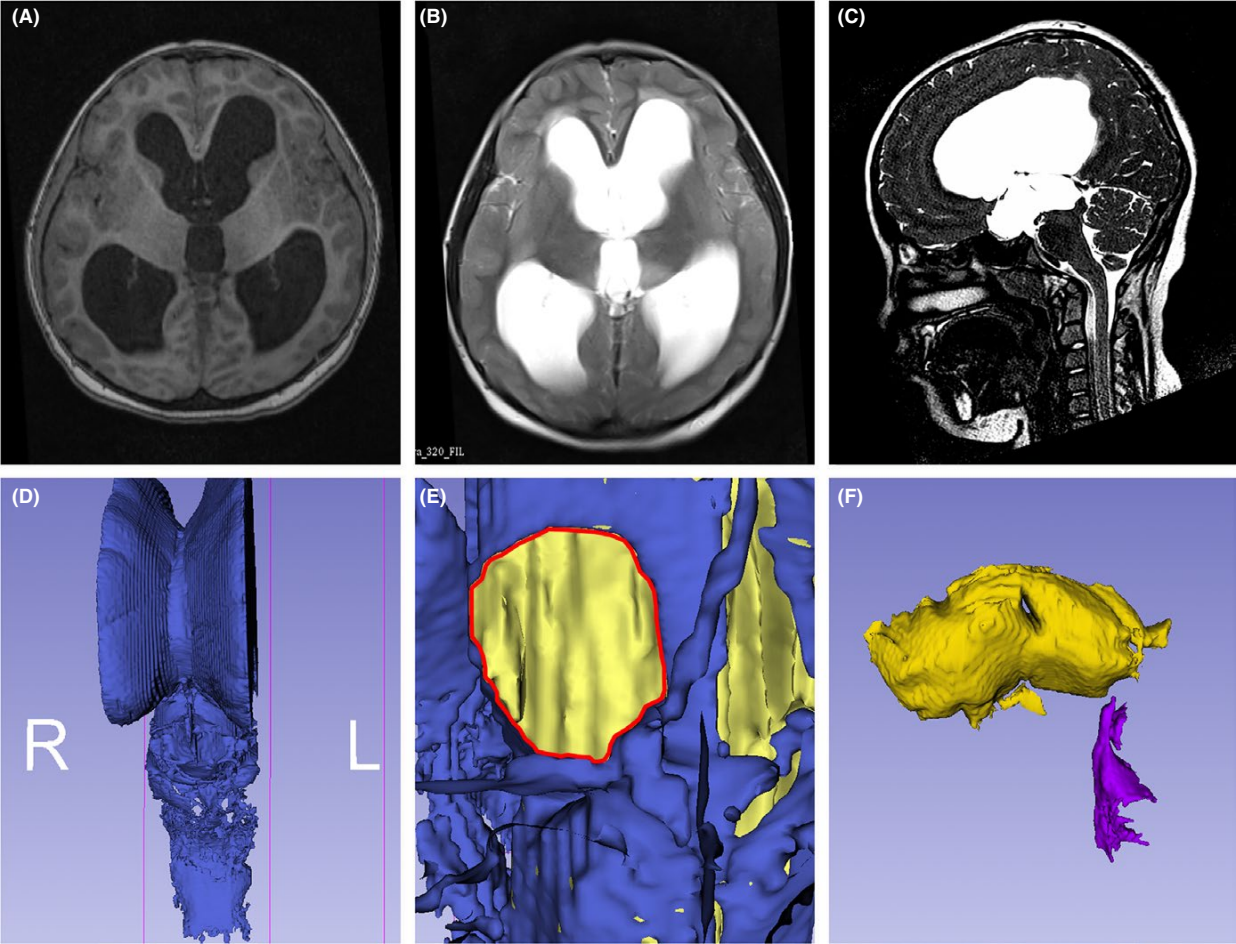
Patient's MRI image and reconstructed virtual endoscopy image of cerebral ventricles. (A and B) T1 and T2 images showed lateral ventricles, and the third ventricle had enlarged, and the surrounding brain tissue was oedematous; (C) 3D‐SPACE image showed that the cerebral aqueduct seemed obstructed; (D) 3D reconstruction of ventricular system with software 3D Slicer; (E) virtual endoscopy image demonstrated that the interventricular foramen (red line) was enlarged; (F) the third ventricle failed to connect to the fourth ventricle because the cerebral aqueduct was obstructed
